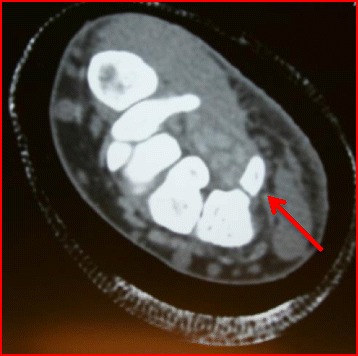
Axial CT image demonstrating a hook of the hamate fracture (*red arrow*) in a college hockey player
